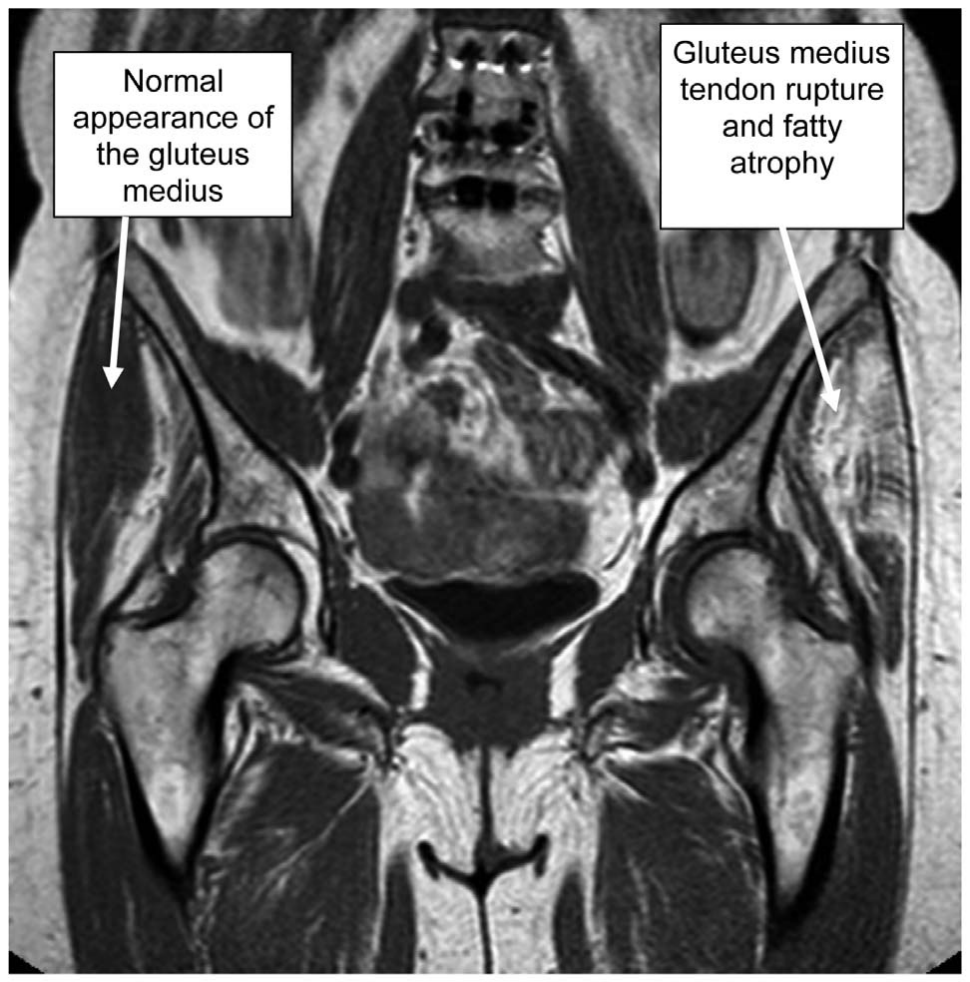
Hip abductor damage on MRI. Shown is the coronal T1-weighted MR-image at the level of the greater trochanter of a 72 year old female patient, complaining of severe lateral hip pain on the left side, radiating down to the knee. The greater trochanter is very tender on palpation and the patient presents with a reduced walking distance and difficulties during the gait cycle. The Hip Lag Sign was positive on the left side.
